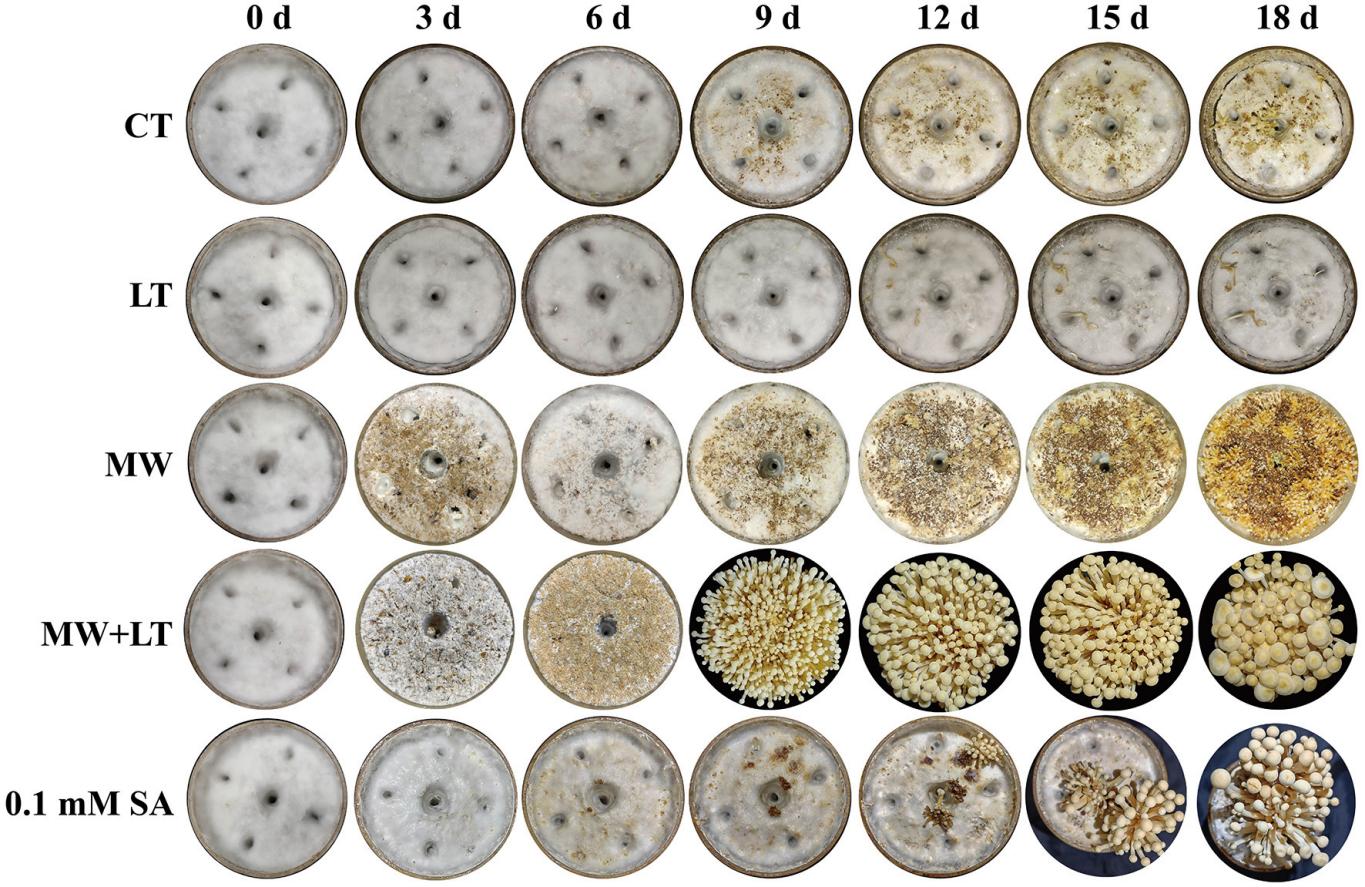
Effects of four treatments, namely LT, MW, MW + LT, and 0.1 mM exogenous SA, on the colony morphology and primordia formation in *F. filiformis*. CT: Control (no treatment), LT, low temperature; MW, mechanical wound; MW + LT, the combination of mechanical wound and low temperature, 0~18 d: 0 to 18 days after four treatments.
